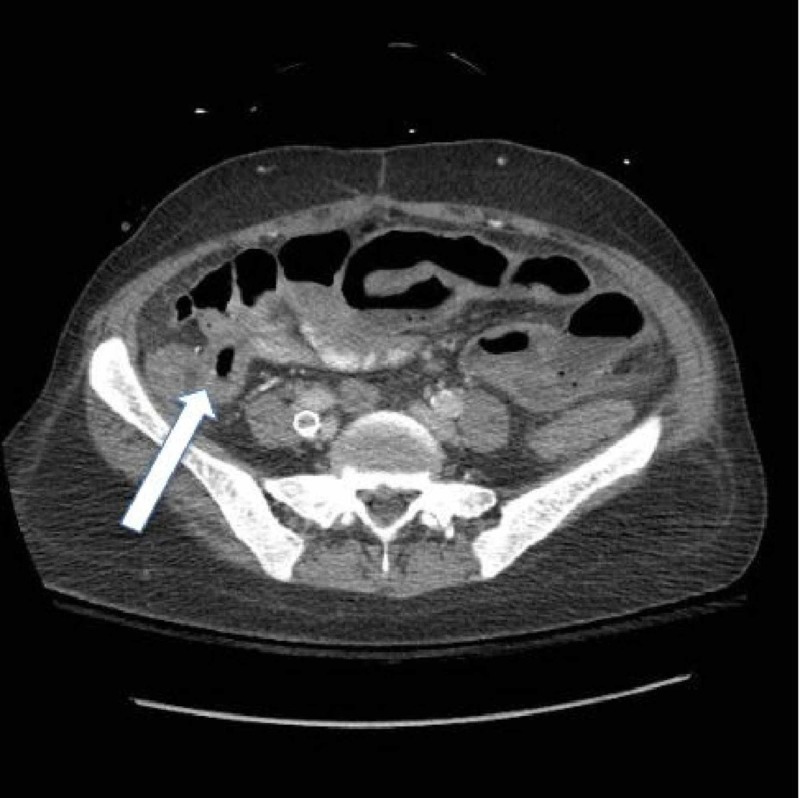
CT of the abdomen and pelvis with contrast findings showing enterocolitis involving the majority of the ileum and proximal ascending colon. These loops also demonstrate decreased enhancement worrisome for ischemia.
